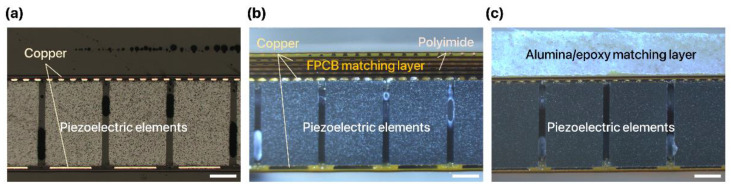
Cross-sectional optical images of 1D linear ultrasound transducer arrays (a) without acoustic matching layer, (b) with the FPCB matching layer, and (c) with the alumina/epoxy matching layer (scale bar: 200 µm).
